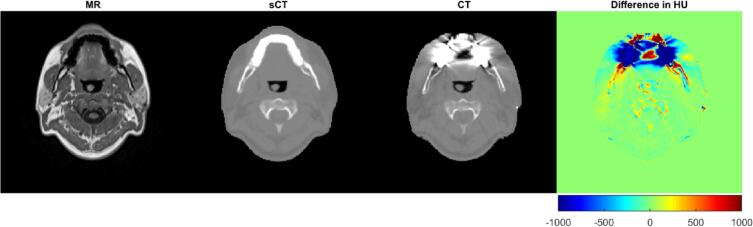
The magnetic resonance image (MRI), generated synthetic computed tomography (sCT) data with minor dental artefacts, computed tomography (CT) data with severe dental artifacts and difference between sCT and CT in Hounsfield units (HU).
